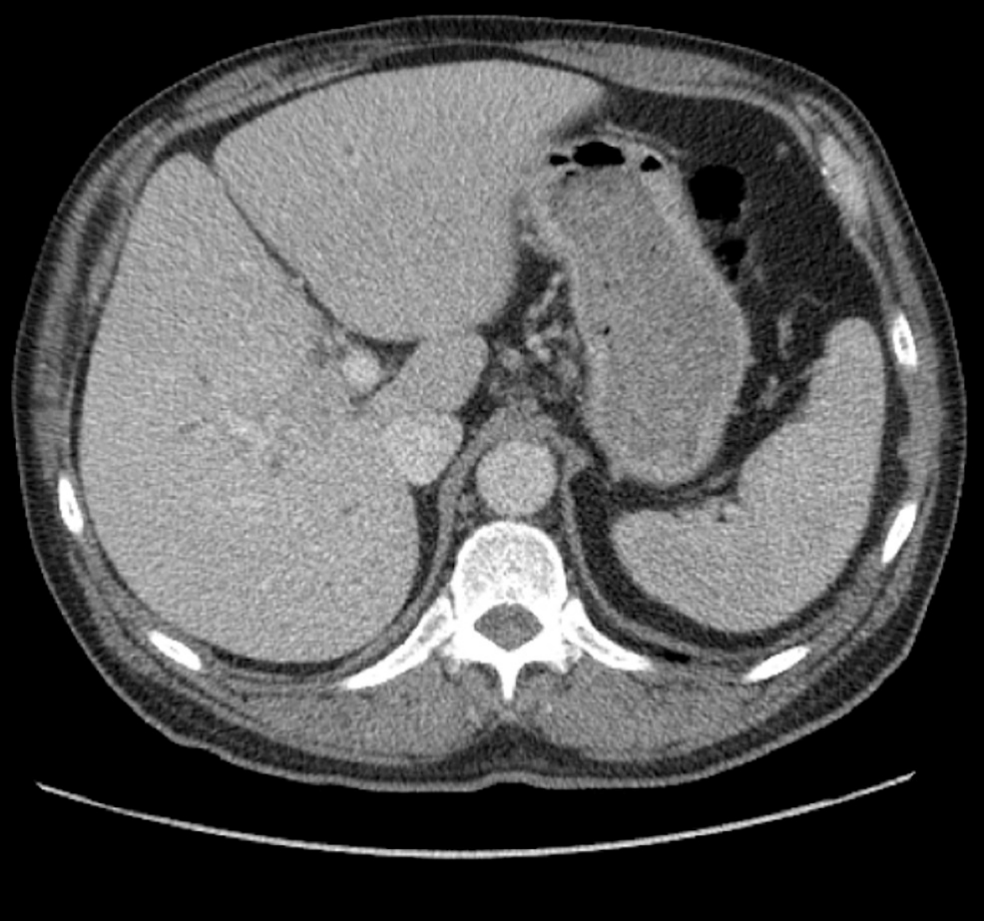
CT showing mild to moderate intrahepatic biliary ductal dilatation with a 4.5 cm x 2.5 cm hilar lesion
